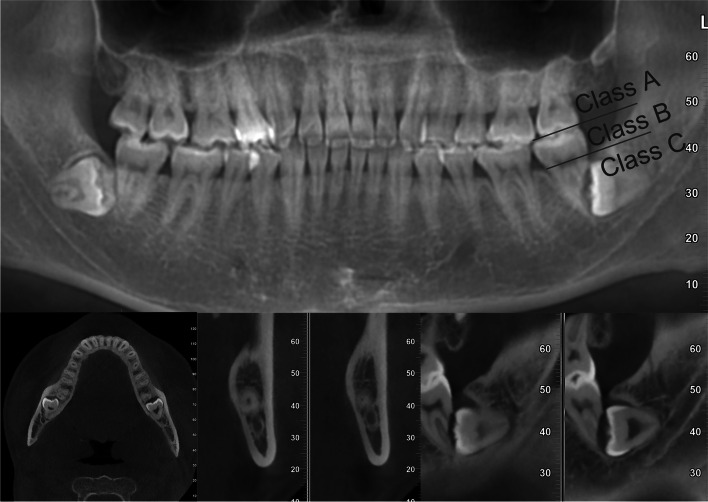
Preoperative CBCT image. #38 was in a horizontal position and the crowns were located below the cervical line of #37
